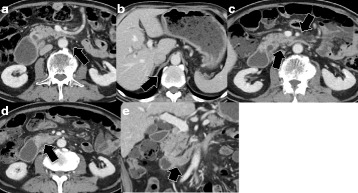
After three courses of chemotherapy, enhanced computed tomography revealed a decrease in the sizes of the para-aortic (a, *arrow*) and paravertebral lymph node (b, *arrow*), regional lymph nodes (c, *arrows*), and primary duodenal tumor (d, e, *arrows*)
